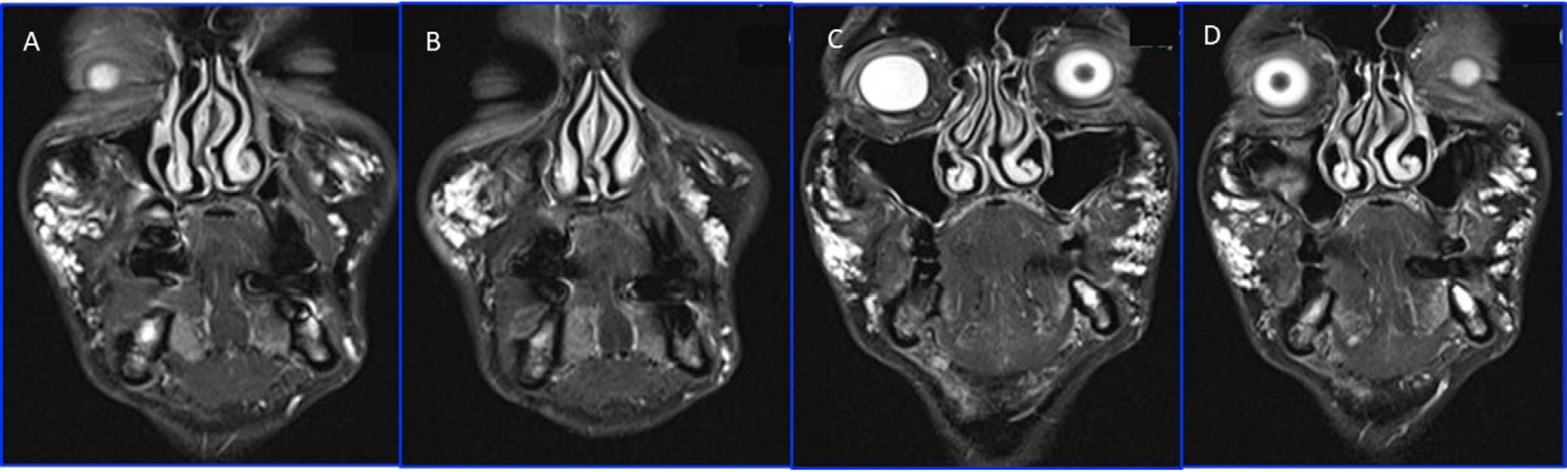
MRI: Coronal T2 TIRM of III MRI, showing HA filler in the zygomatic and cheek region.
